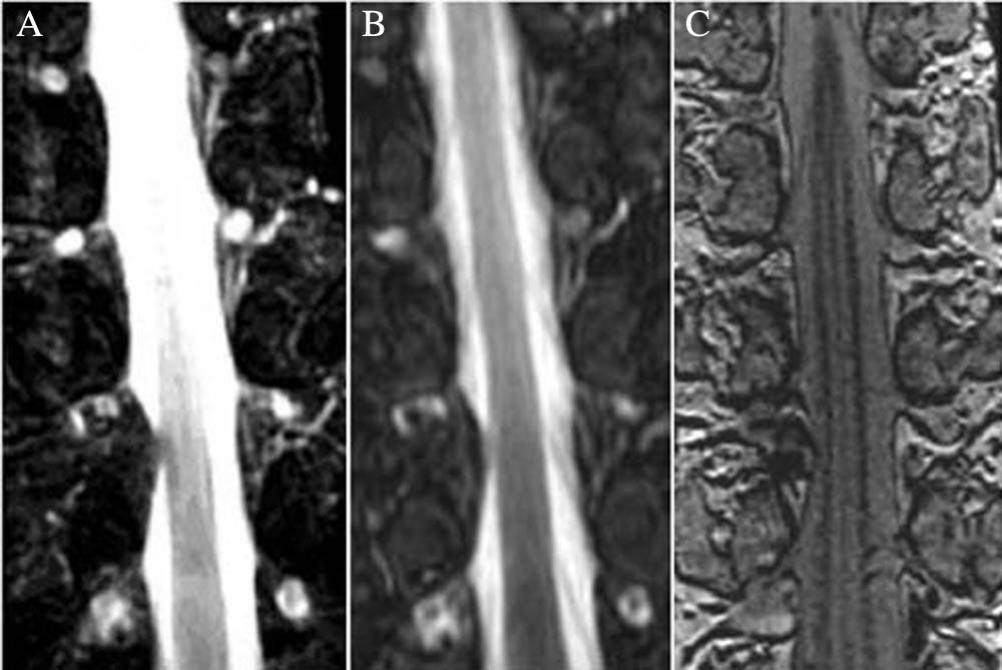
Different MRI sequences show DRG locations. (A) FSE-STIR T2WI; (B) Fs-FRFSE T2WI; (C) 3D FIESTA-c.
